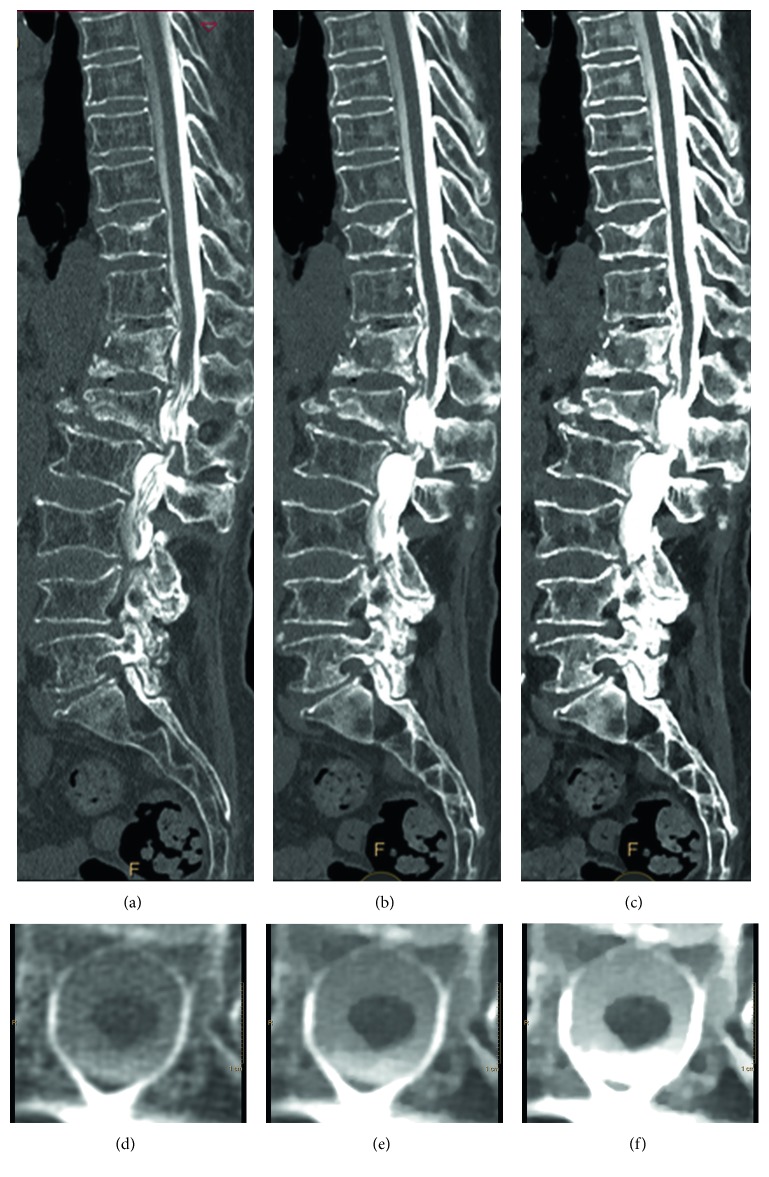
Computed tomography (CT) myelography images from a 73-year-old man (body weight 56 kg) with severe lumbar canal stenosis at the level of L1-L2 and multiple compression fractures (T10, T12, and L1). (a) Sagittal conventional CT myelography image. (b) Sagittal virtual monochromatic image (55 keV). (c) Sagittal virtual monochromatic image (40 keV). (d) Axial conventional CT myelography image at T6. (e) Axial virtual monochromatic image (55 keV). (f) Axial virtual monochromatic image (40 keV). The window setting was as follows: window width, 1250 Hounsfield units; window level, 250 Hounsfield units.
